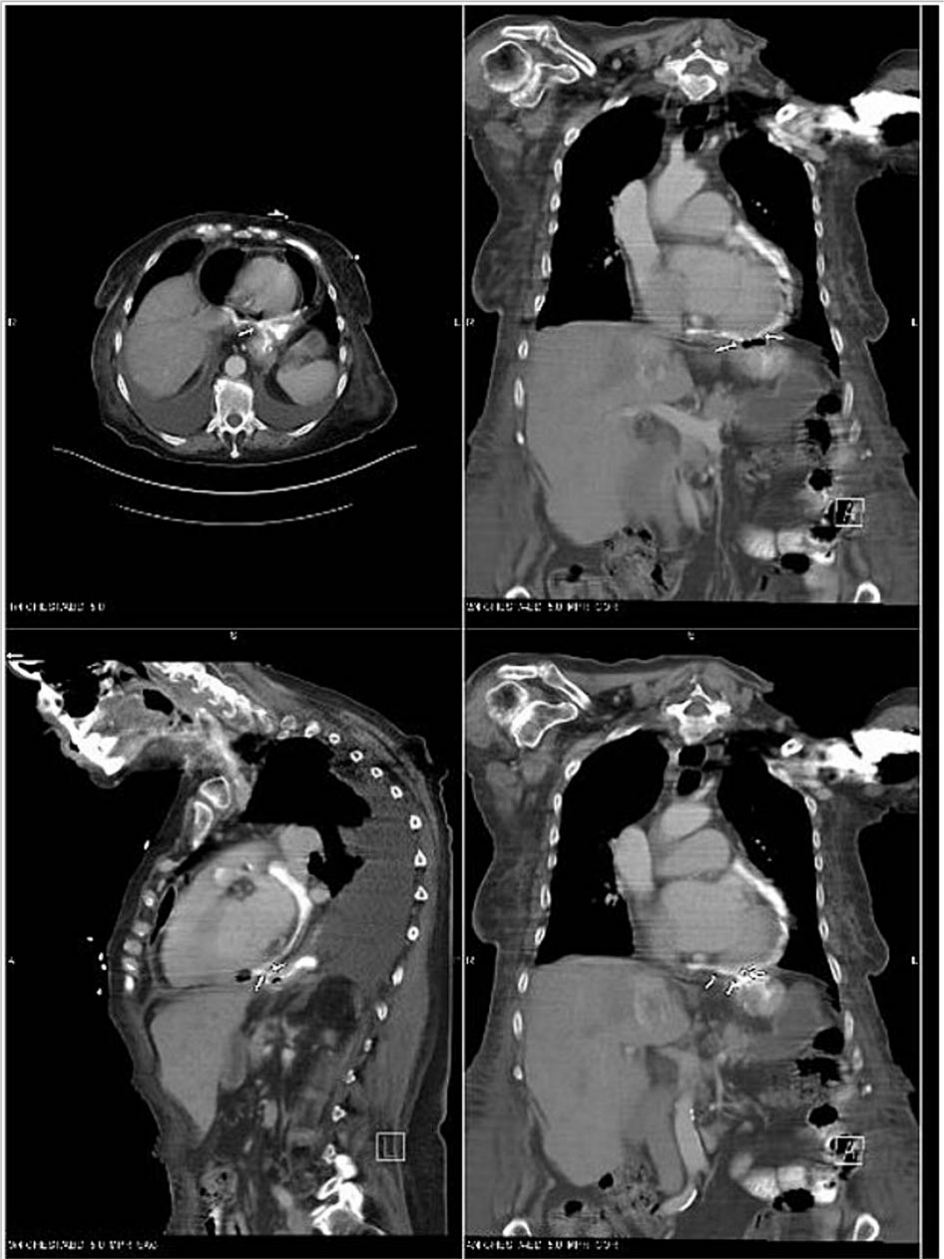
CT Chest with oral contrast demonstrating gastropericardial fistula.
